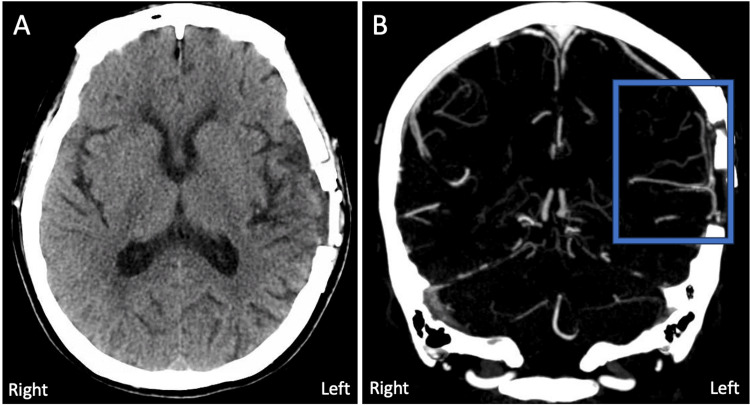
CT head imaging at the 5-month follow-up A) Axial CT non-contrast imaging depicting resolution of postoperative air. B) Coronal CT angiography demonstrating STA-MCA bypass. The blue box focuses on the site of anastomosis CT: computed tomography; STA: superficial temporal artery; MCA: middle cerebral artery
